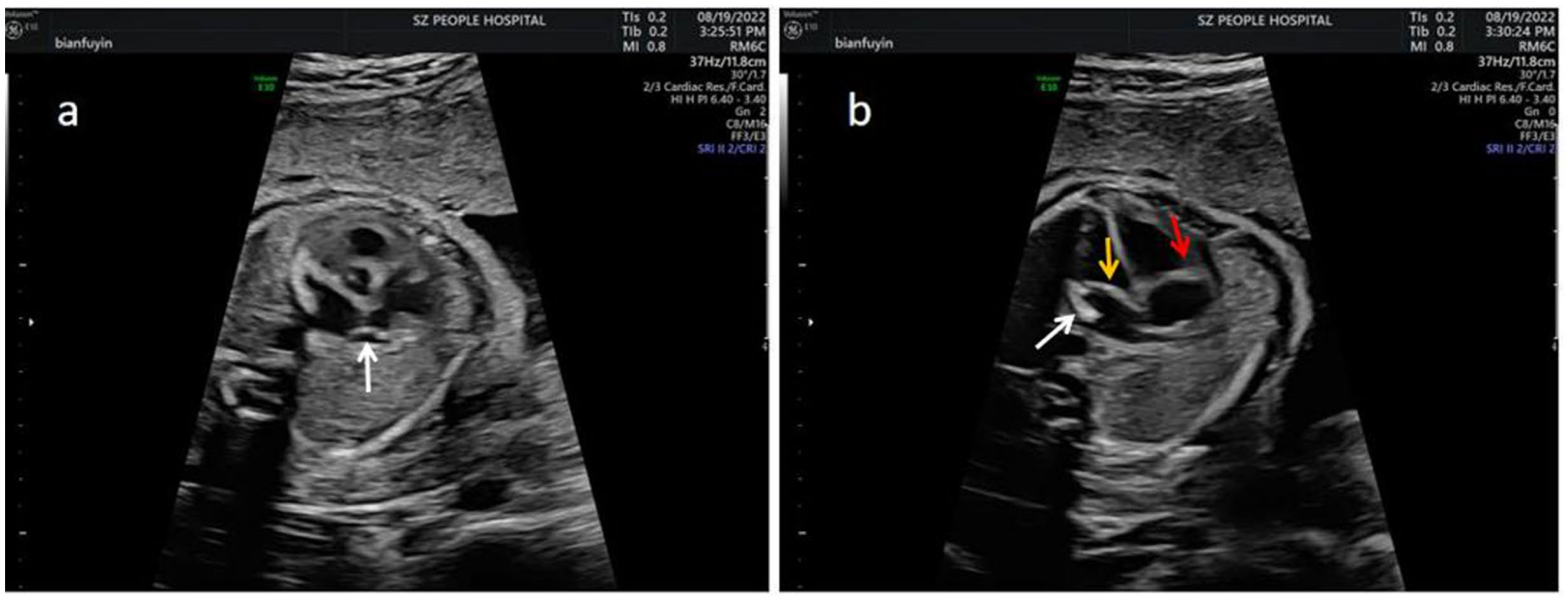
The echocardiographic features of isolated mild EFE in different site. (a) Left atrial appendage (white arrow). (b) MV (yellow arrow), TV (red arrow) and left atrial side wall (white arrow).
